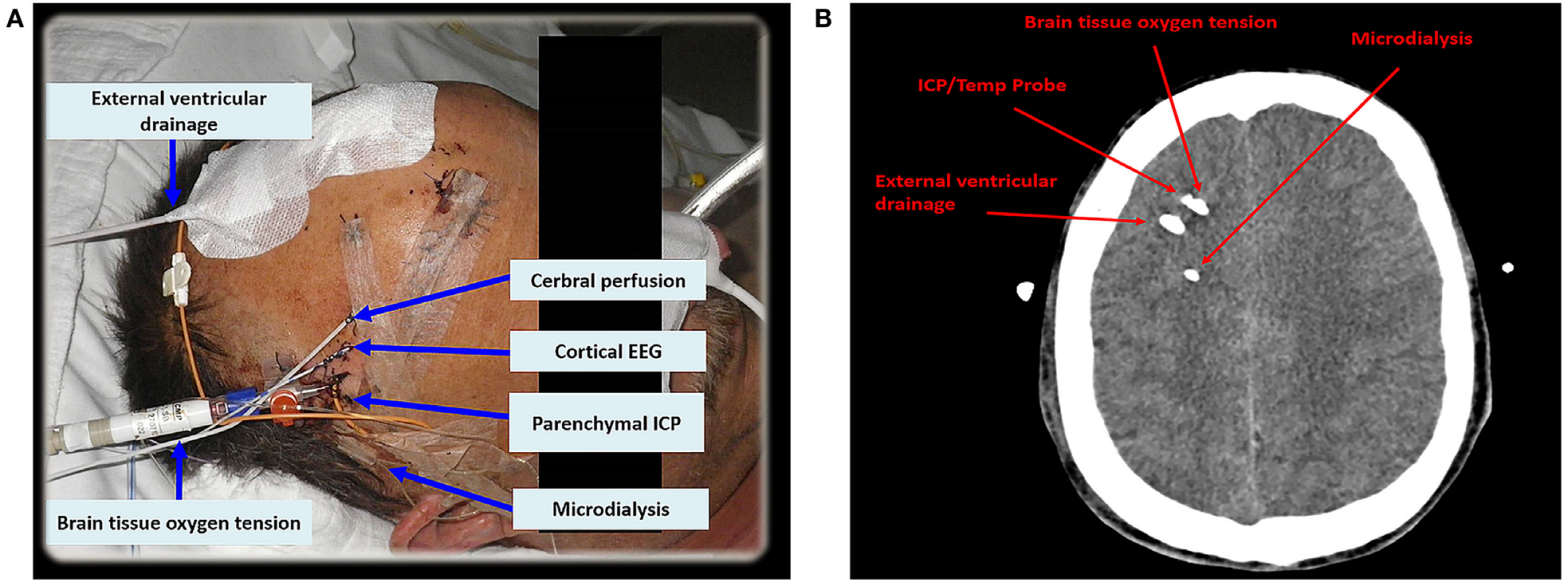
In (A), multimodal neuromonitoring catheters are tunneled in a patient with subarachnoid hemorrhage. (B) Shows neuromonitoring catheters placed in the white matter on an axial computed tomography. EEG, electroencephalography; ICP, intracranial pressure; Temp, temperature.
